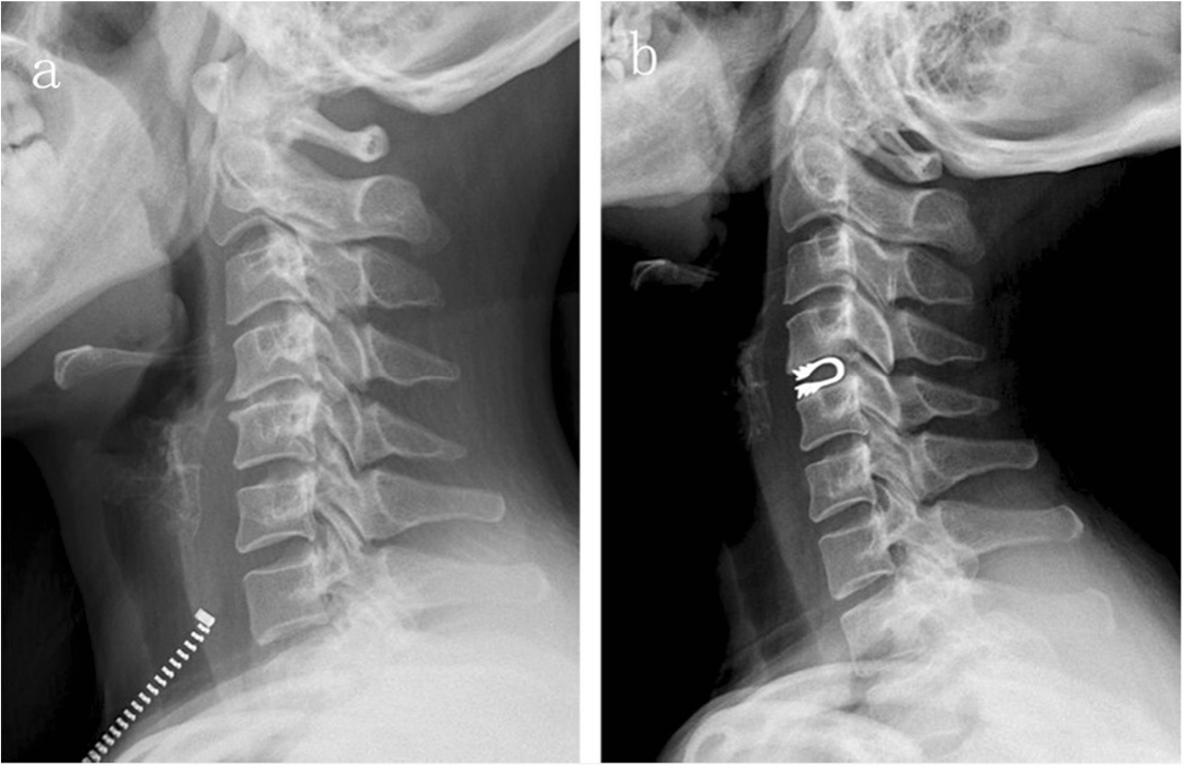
Preoperation and postoperation of DCI. a Preoperative lateral X-rays showed the height of C4-5 decreased, and kyphosis existed. b Postoperative lateral X-rays showed the kyphotic curvature retrieved.
